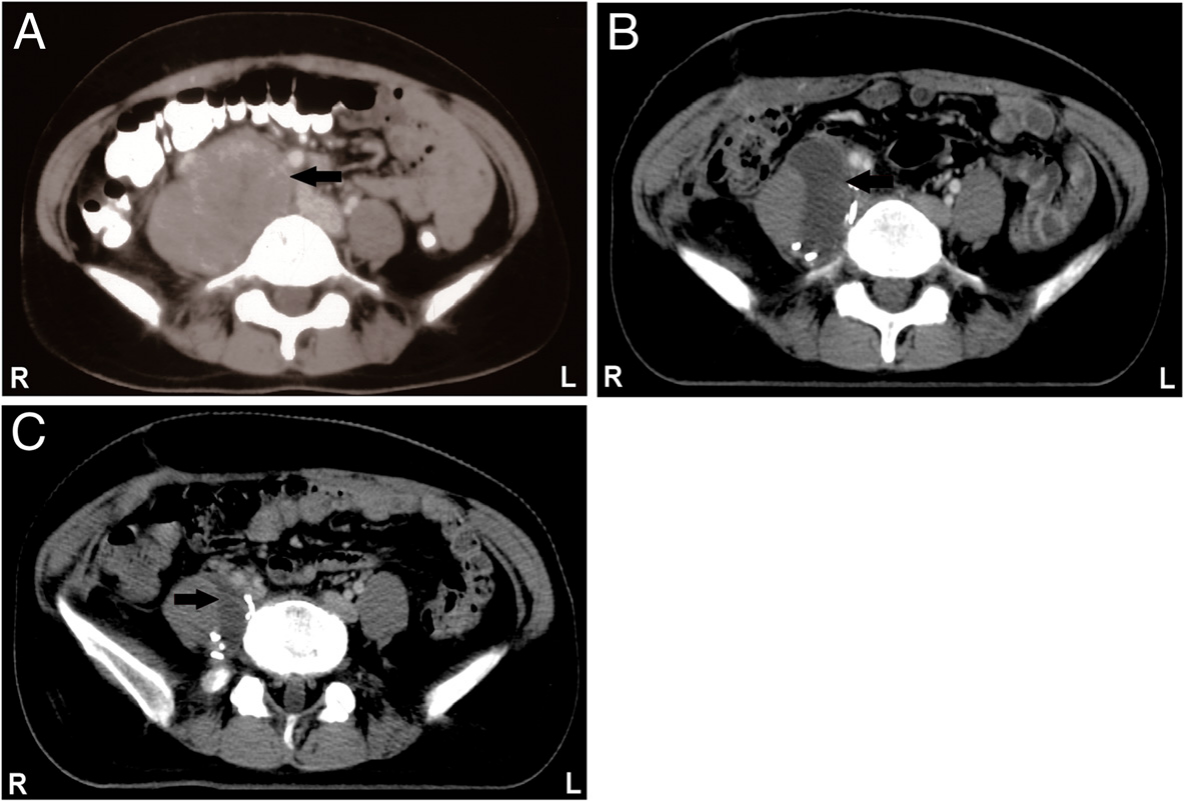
Computed tomography images of retroperitoneal follicular dendritic cell sarcoma. A: Contrast-enhanced computed tomography scan of the abdomen revealing an 8.6cm retroperitoneal mass (arrow); B: liquid dark area (arrow) observed in the surgical area after operation; C: significant shrinking of the liquid dark area (arrow) was observed after adjuvant therapy.
